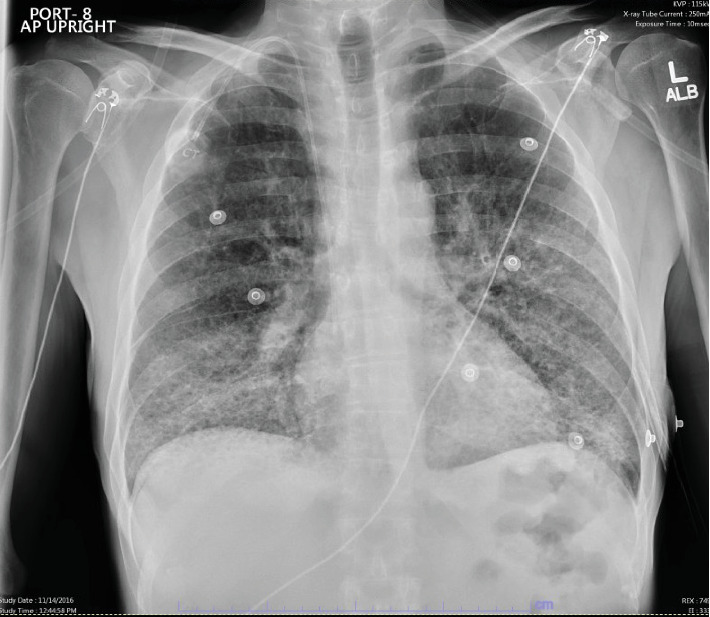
Chest radiograph upon initial presentation to the emergency department. Bilateral interstitial and alveolar airspace disease, left greater than right. Appearance is most suggestive of multilobar pneumonia. This does not have the typical appearance of CHF. Pulmonary hemorrhage or other infectious/inflammatory processes are also on the differential.
